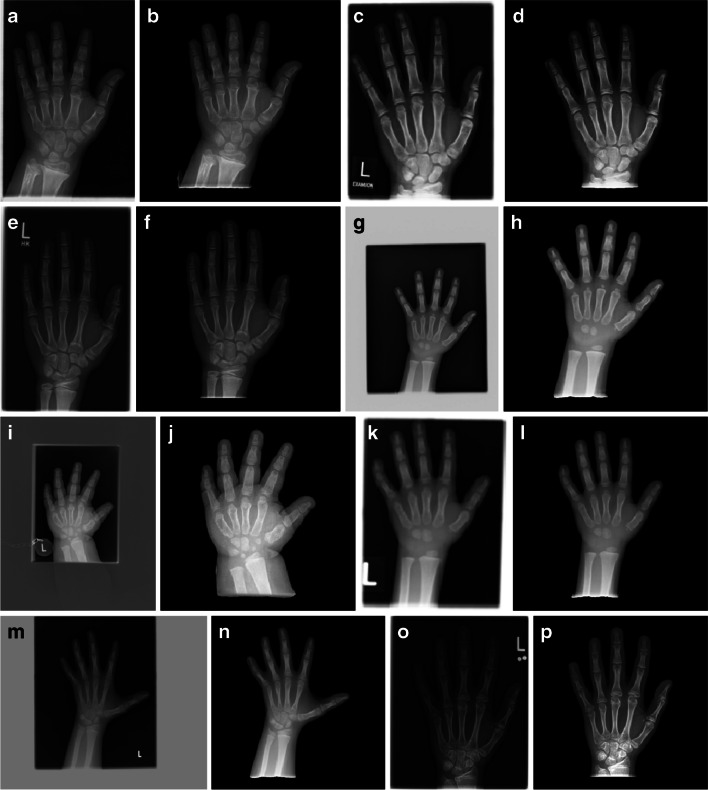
Example dorsopalmar hand and wrist radiographs for each bone dysplasia in the German Dysplasia Bone Dataset. a, b A 10-year-old boy with achondroplasia. c, d A 10-year-old girl with hypochondroplasia. e, f A 12-year-old boy with intrauterine growth restriction. g, h A 3-year-old boy with Noonan syndrome. i, j A 4-year-old girl with pseudohypoparathyroidism. k, l A 4-year-old boy with a mutation of the *SHOX* gene. m, n A 9-year-old girl with Silver-Russell syndrome. o, p A 14-year-old girl with Ullrich-Turner syndrome. For each example, the original raw (a, c, e, g, i, k, m, o) and preprocessed (b, d, f, h, j, l, n, p) image is shown. There is a wide range of relative scales within the images; image qualities vary and show artifacts such as labels and white or gray backgrounds caused by scanning
